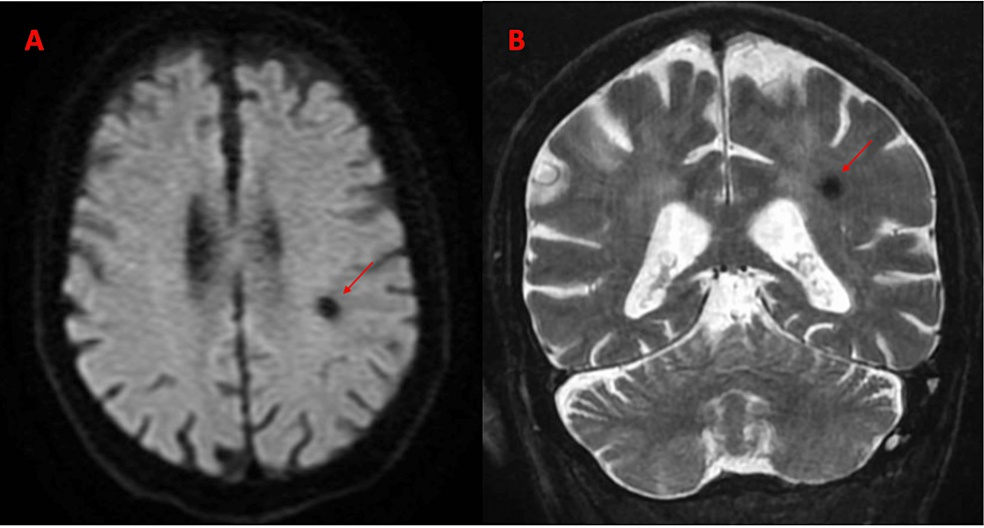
MRI with and without Contrast findings suggest no acute ischemic infarct but a focal 8 mm T2 hypointense lesion in the left posterior frontal lobe representing a calcified hematoma (shown by red arrows). It also showed nonspecific multiple T2/FLAIR hyperintense foci in the supratentorial white matter, likely due to chronic small vessel ischemia. A: Axial Diffusion-weighted imaging, B: Coronal T2 Image.
